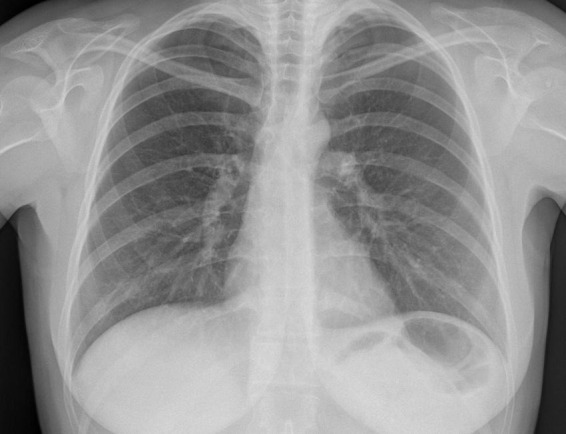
Chest X-ray done at the beginning of IVF treatment.
